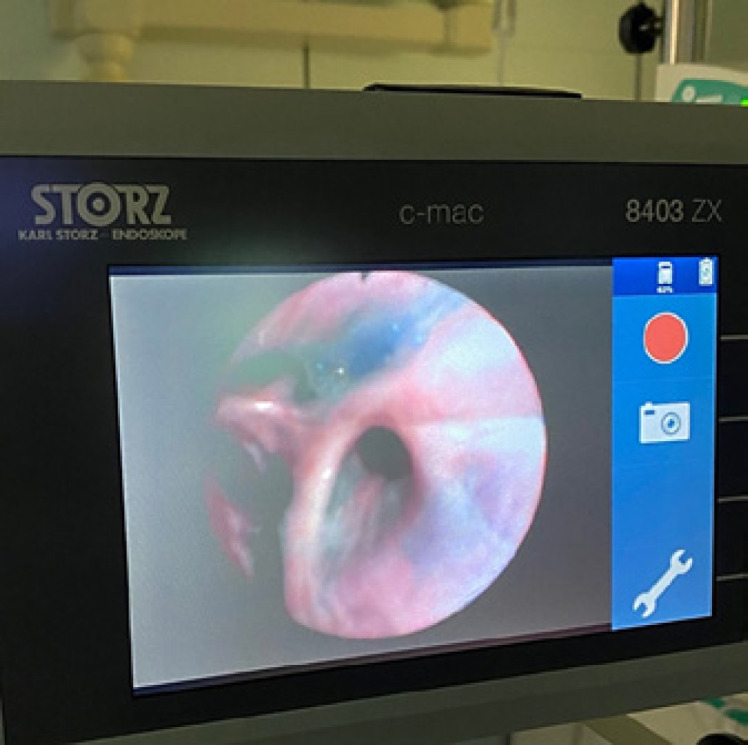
CT scan with oral contrast showing the EPF with intrapulmonary contrast.
